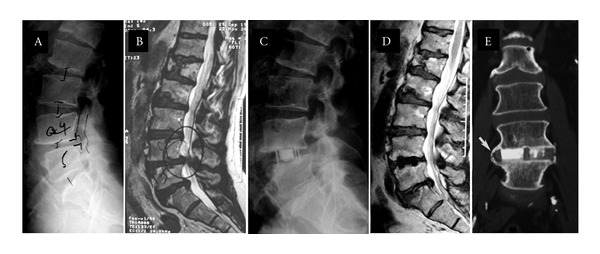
Case example #2. (a) Preoperative X-ray (b) Preoperative MRI sagittal reconstruction (c) 12-month X-ray (d) 24-month MRI sagittal reconstruction (e) 24-month computed tomography sagittal reconstruction. Arrow shows sentinel sign around cage.
